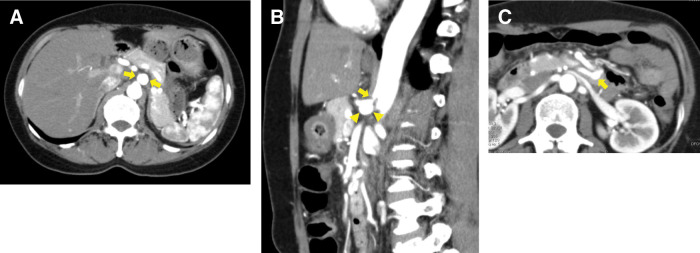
Preoperative contrast-enhanced CT findings. (A) Axial view showing a 15-mm CA aneurysm (arrows). (B) Sagittal view showing severe stenosis at the origin of the CA (arrow) and poststenotic aneurysm (arrowheads). (C) Axial view showing a 7-mm aneurysm of the inferior PDA (arrow). CA, celiac artery; PDA, pancreaticoduodenal artery
